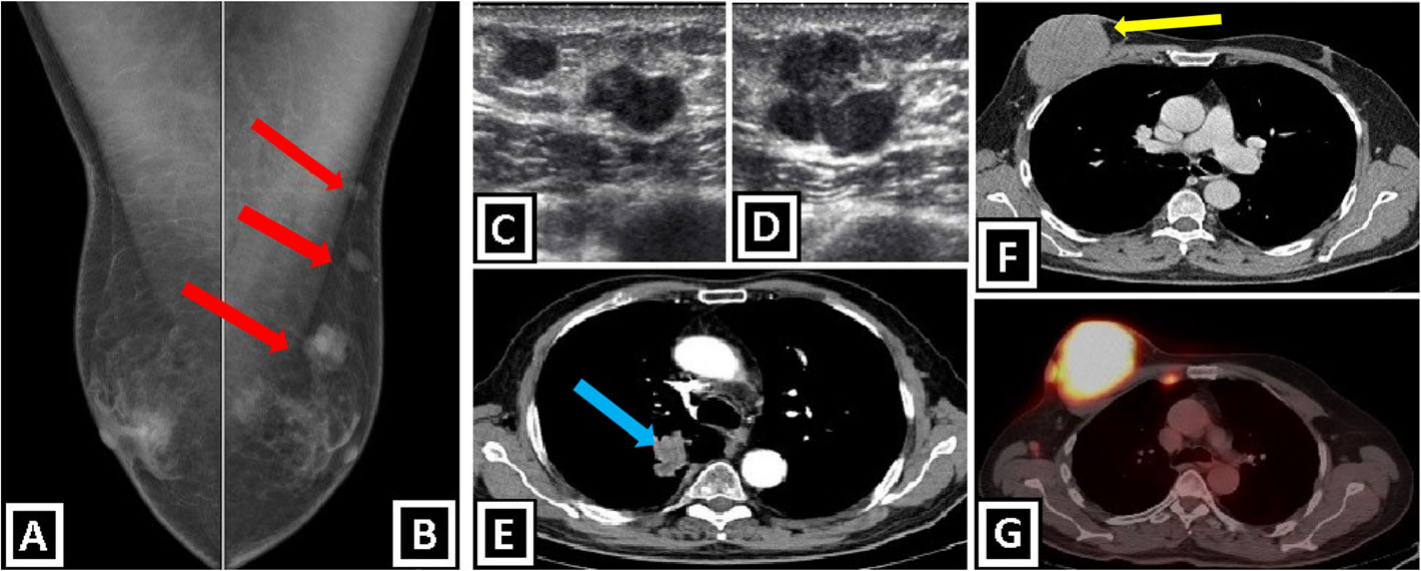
Secondary malignant breast masses in males. a–e Radiological images of a 58-year-old man with lung adenocarcinoma. a, b Bilateral medio-lateral oblique mammograms show bilateral gynecomastia and three round masses in left breast (red arrows). c, d US images demonstrate macrolobulated round hypoechoic metastatic masses. e Contrast-enhanced axial CT image shows lung adenocarcinoma in left lung (blue arrow). f, g Breast involvement of diffuse large B cell lymphoma in a 63-year-old patient. Contrast enhanced axial CT image shows a circumscribed mass within left breast (f). PET-CT scan reveals FDG-avid masses which are consistent with lymphamatous infiltration located in not only right breast, but also right internal mammarian and axillary lymph node stations (g)
